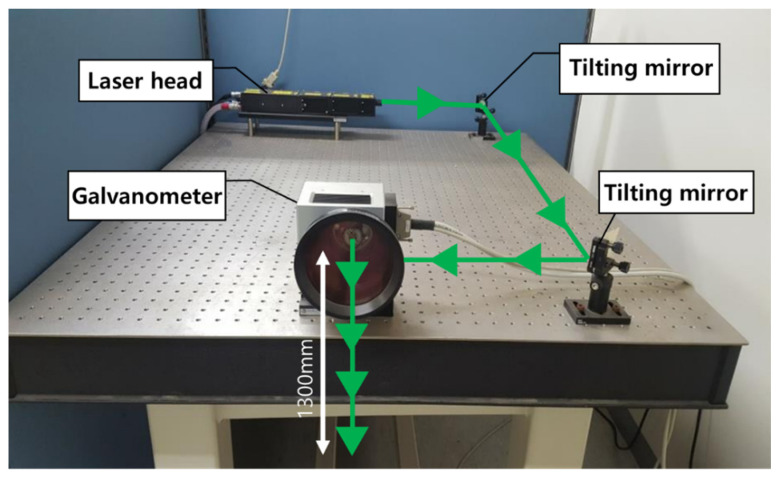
A noncontact laser ultrasonic scanning system composed of a Q-switched Nd:YAG pulsed laser with a galvanometer for ultrasonic excitation scanning [5].
